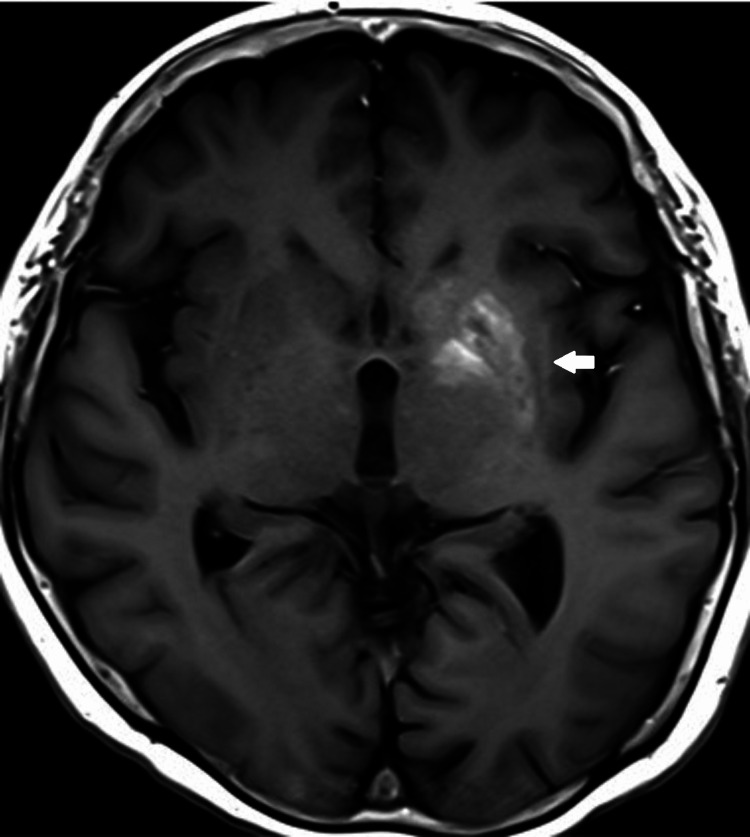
MRI Brain - Intrinsic T1W hyperintensity of the left basal ganglia
